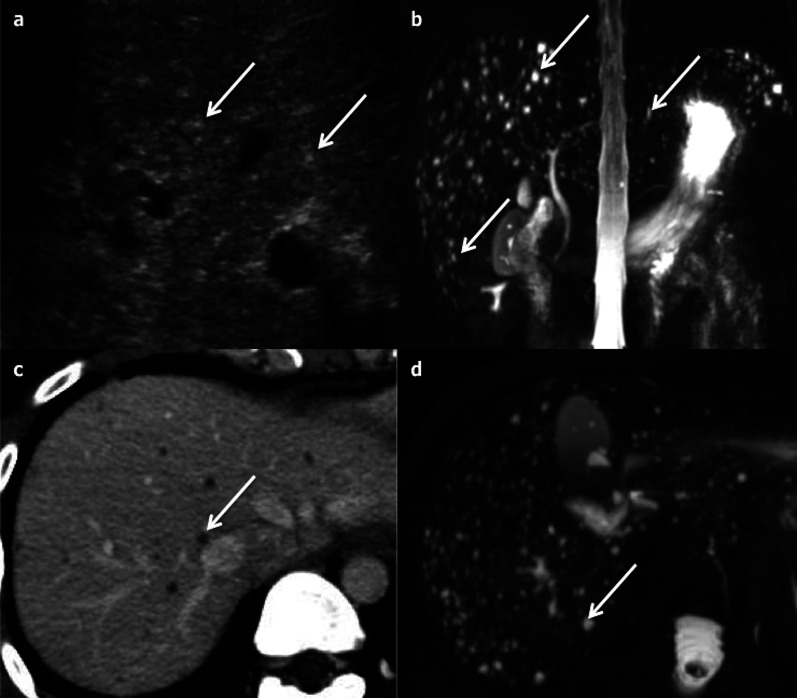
Bile duct hamartomas in a 52-year-old female. a Multiple hyperechoic round nodules (arrows) at ultrasonography, displaying a “snowstorm” pattern. b Coronal magnetic resonance cholangiopancreatography shows several small hyperintense nodules, which do not communicate with the biliary tract (arrows). c Axial computed tomography on portal venous phase shows bilobar small round-shaped hypoattenuating nodules, which can be better detected with an axial magnetic resonance cholangiopancreatography sequence (d)
